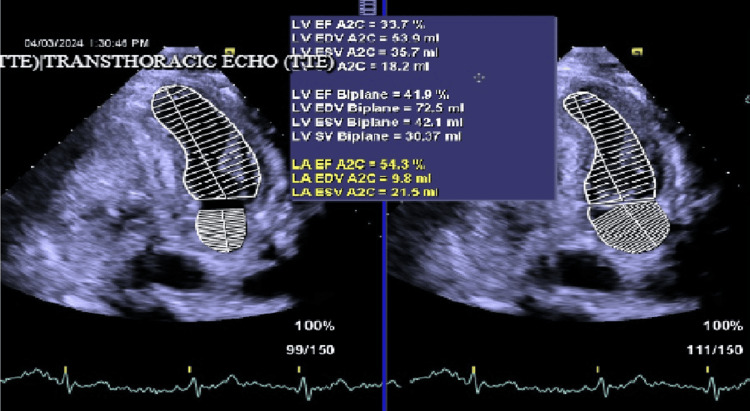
Transthoracic echocardiogram showing EF LV, left ventricular; LA, left atrium; EF, ejection fraction; EDV, end-diastolic volume; ESV, end-systolic volume
